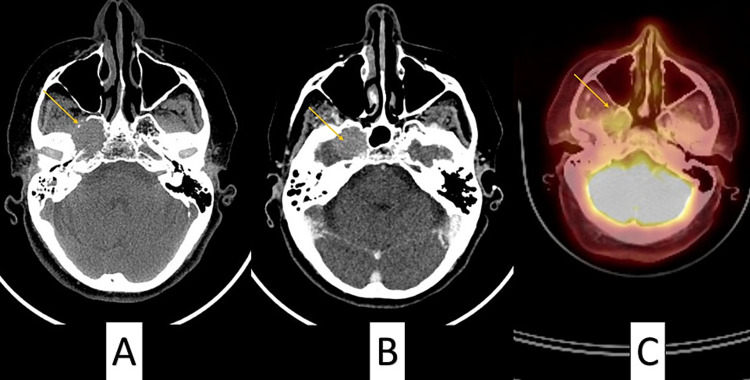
(A, B) Destructive solid heterogeneously enhancing mass of 2.9x2.4 cm (yellow arrow) centered in the medial portion of the right middle skull base involving greater wing of sphenoid, lateral sphenoid body, and pterygoid plates causing bony erosion of petrous segment. (C) PET CT showing mildly enhancing right skull base lesion in the same area as the location of the mass.
